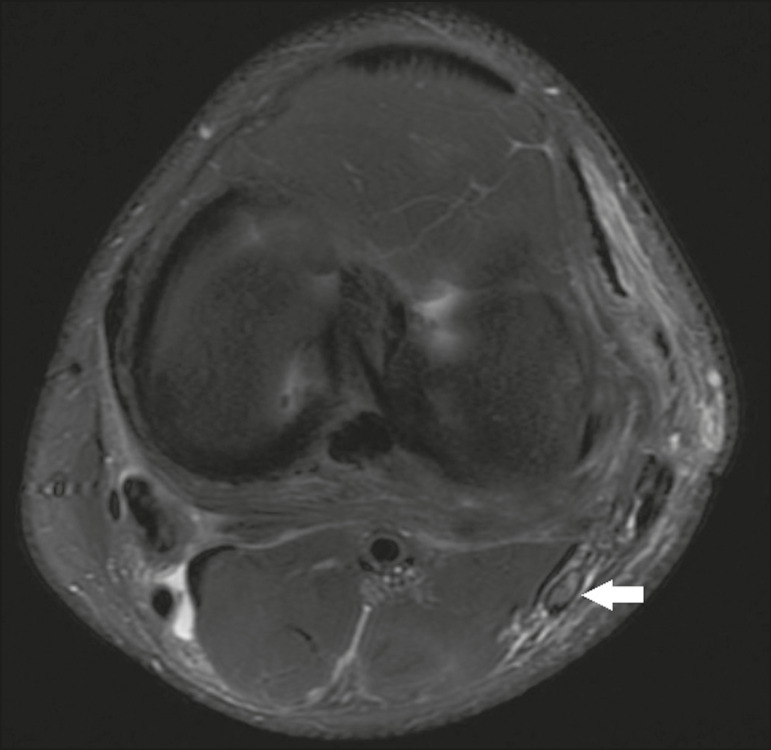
Axial T2-weighted MRI sequence with fat saturation, showing CPN axonotmesis. Note the increased signal intensity, clearly increased dimensions, and poorly defined fascicles in the CPN (arrow).
